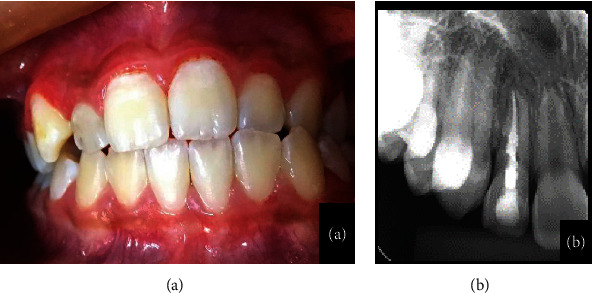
(a, b) Buccal gingival tissue of the maxillary lateral incisor reveals normal clinical features. Initial periapical radiography shows sealing material in the pulp cavity.
